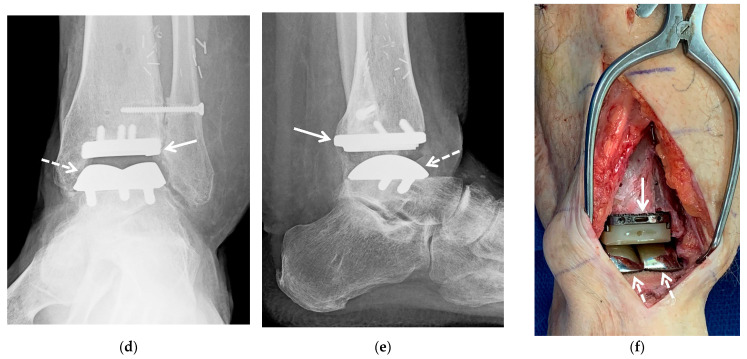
(a–f) A 36-year-old male with posttraumatic osteoarthritis of the left tibiotalar joint and equinus contracture resulting from open distal tibia fracture treated with open reduction internal fixation and free flap, with previous hardware removal, subsequently treated with ankle arthroplasty and gastrocnemius resection. (a) Mortise and (b) lateral weight-bearing radiographs of the left ankle show marked asymmetric narrowing of the tibiotalar joint (white arrows) with marginal osteophytes and intraarticular bodies consistent with severe osteoarthritis. Note tracts in the calcaneus and talus related to removed surgical hardware and retained laterally placed screw transfixing the distal tibiofibular syndesmosis. Numerous vascular clips overly the soft tissues of the lower leg. (c) Coronal fluid sensitive MR image shows asymmetric narrowing of the tibiotalar joint (white arrows). (d) Mortise and (e) lateral postoperative radiographs show interval placement of total ankle arthroplasty (Wright Medical Infinity with an Inbone talus). Note well seated tibial (white arrow) and talar (dashed white arrow) prosthesis components. (f) The intraoperative image shows tibial (white arrow) and talar components (dashed white arrow) in place.
